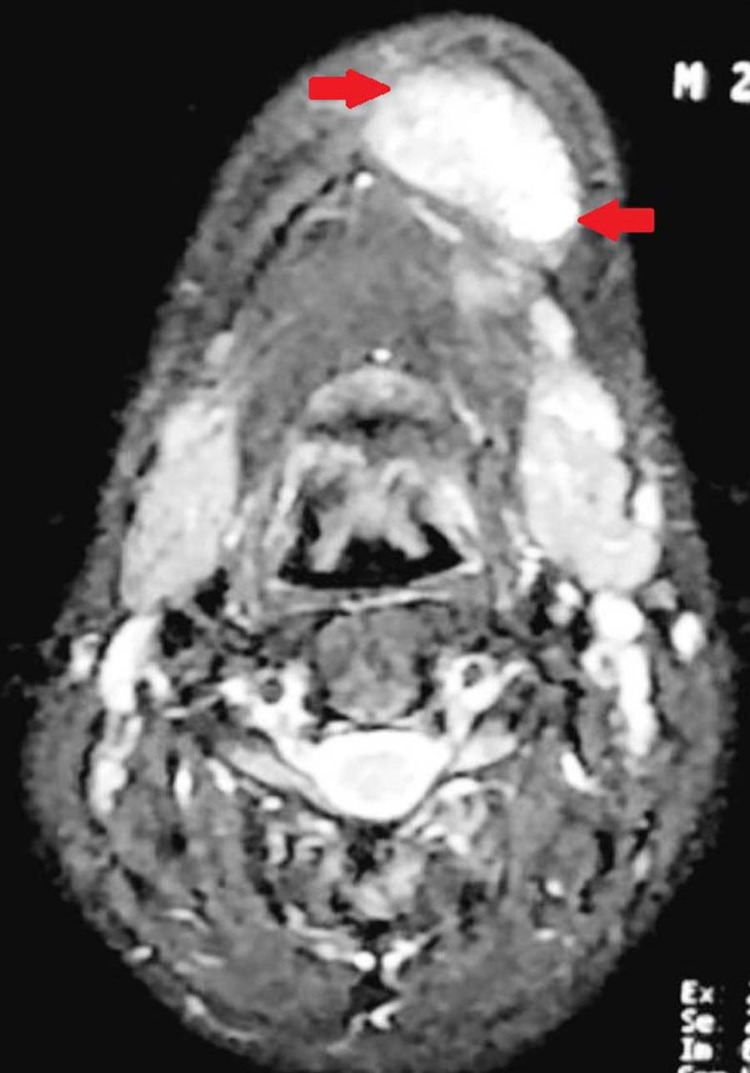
Brown tumor evidenced on MRI localized to the patient's mandible MRI: Magnetic Resonance Imaging
